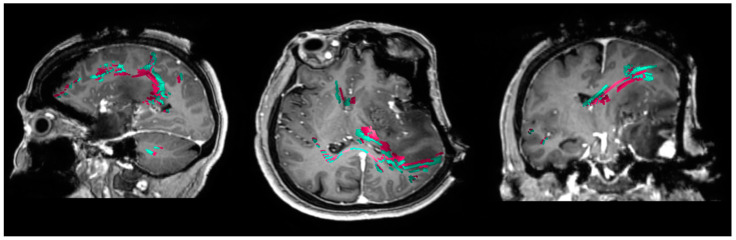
nTMS-based language tractography corrected by IBEF in an ioMRI scan. This figure shows motor fiber tracts based on navigated transcranial magnetic stimulation (nTMS) in an intraoperative magnetic resonance imaging (ioMRI) scan in an exemplary patient case. The preoperative motor fiber tracts are shown in pink. The fiber tracts corrected by ioMRI-based elastic fusion (IBEF) are shown in cyan.
